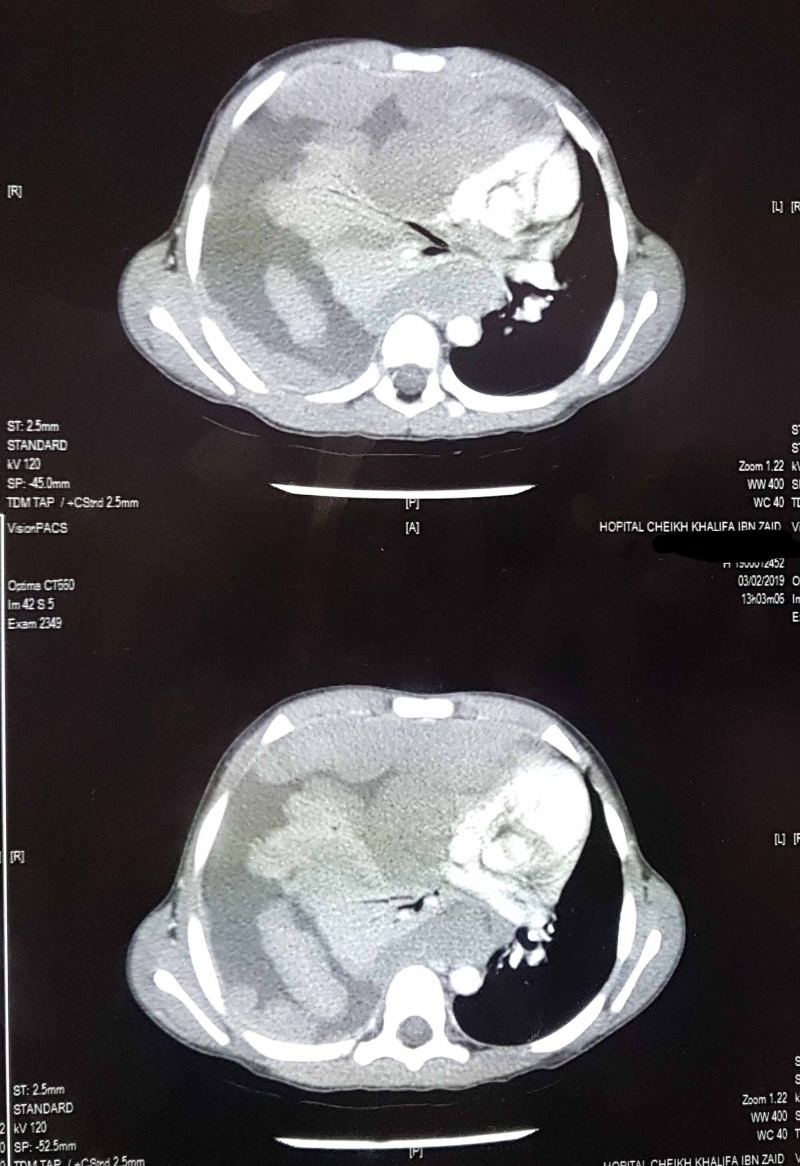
Axial CT sections of the thorax: Right pleurisy with polylobed intra-pleural masses
